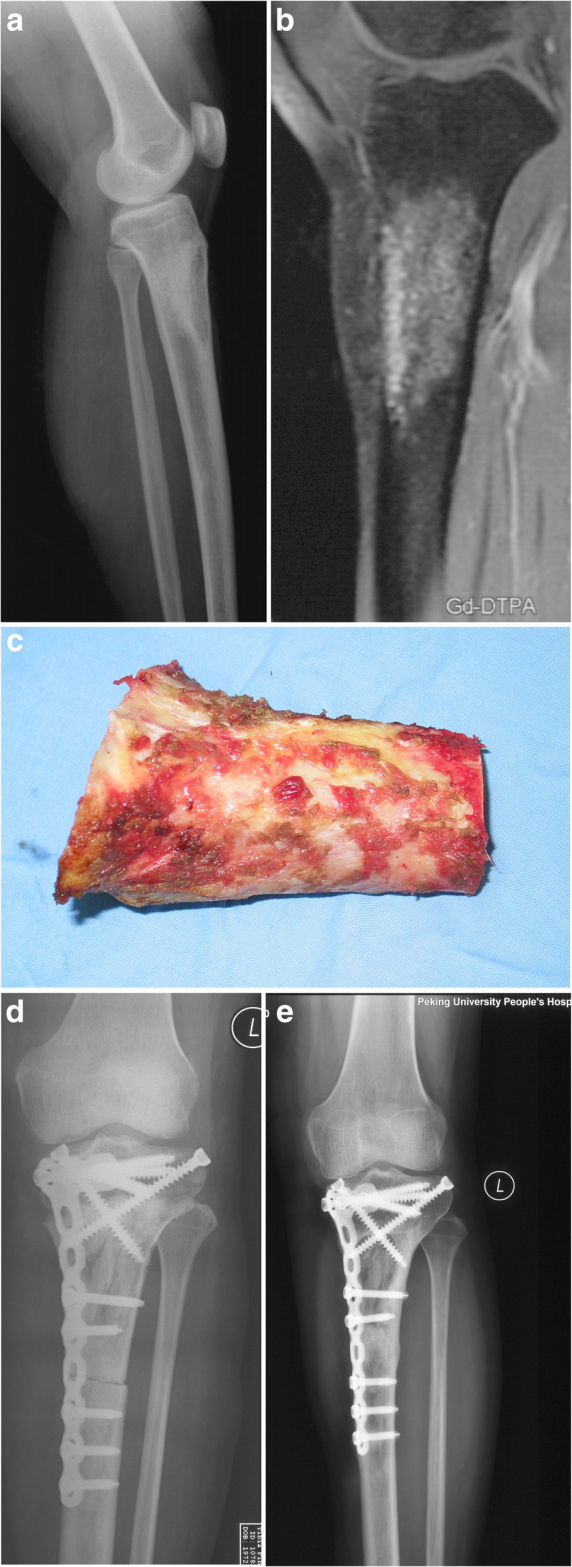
A 42-year-old patient with an adamantinoma of the left proximal tibia. a X-ray showed the lesion located at the proximal part of the left tibia. b MRI showed the knee joint was not involved by the tumor. c The structural bone and the periosteum were preserved after the tumor was cleared away. d X-ray showed the devitalized bone matched well with the host bones after the operation. e X-ray showed the cutting lines was disappeared, and the devitalized bone integrated well with the host bone at each ends 11 months after the operation
